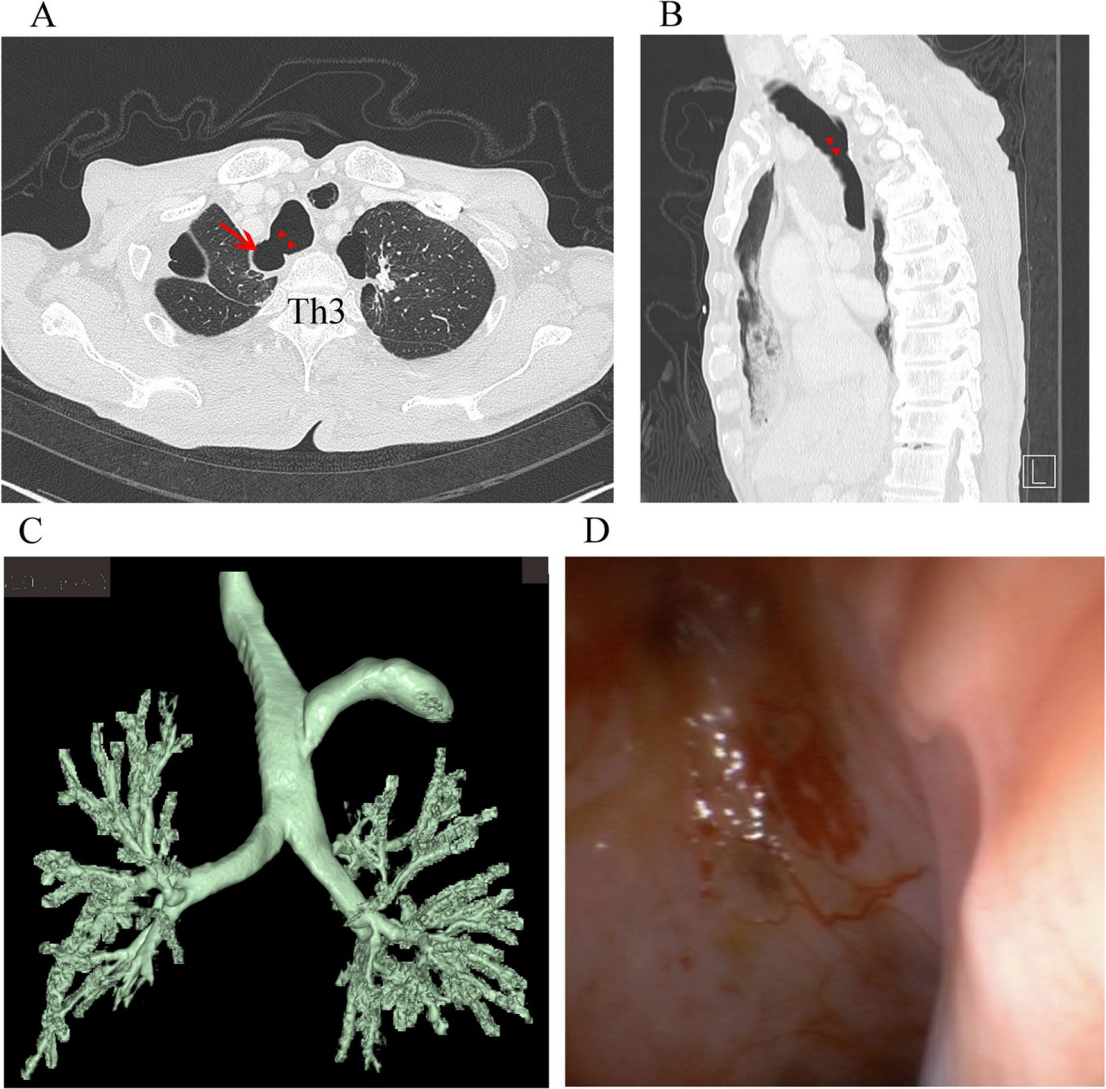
CT findings 6 months after receiving conservative treatment. Axial view at Th3 level (A), sagittal view (B), and 3D-CT reconstruction image (C). The area of defective posterior wall of the trachea (arrowheads) and the dead space (arrow) were significantly decreased. Bronchoscopy showed complete epithelialization of the trachea (D). *Th* thoracic vertebra, *CT* computed tomography
